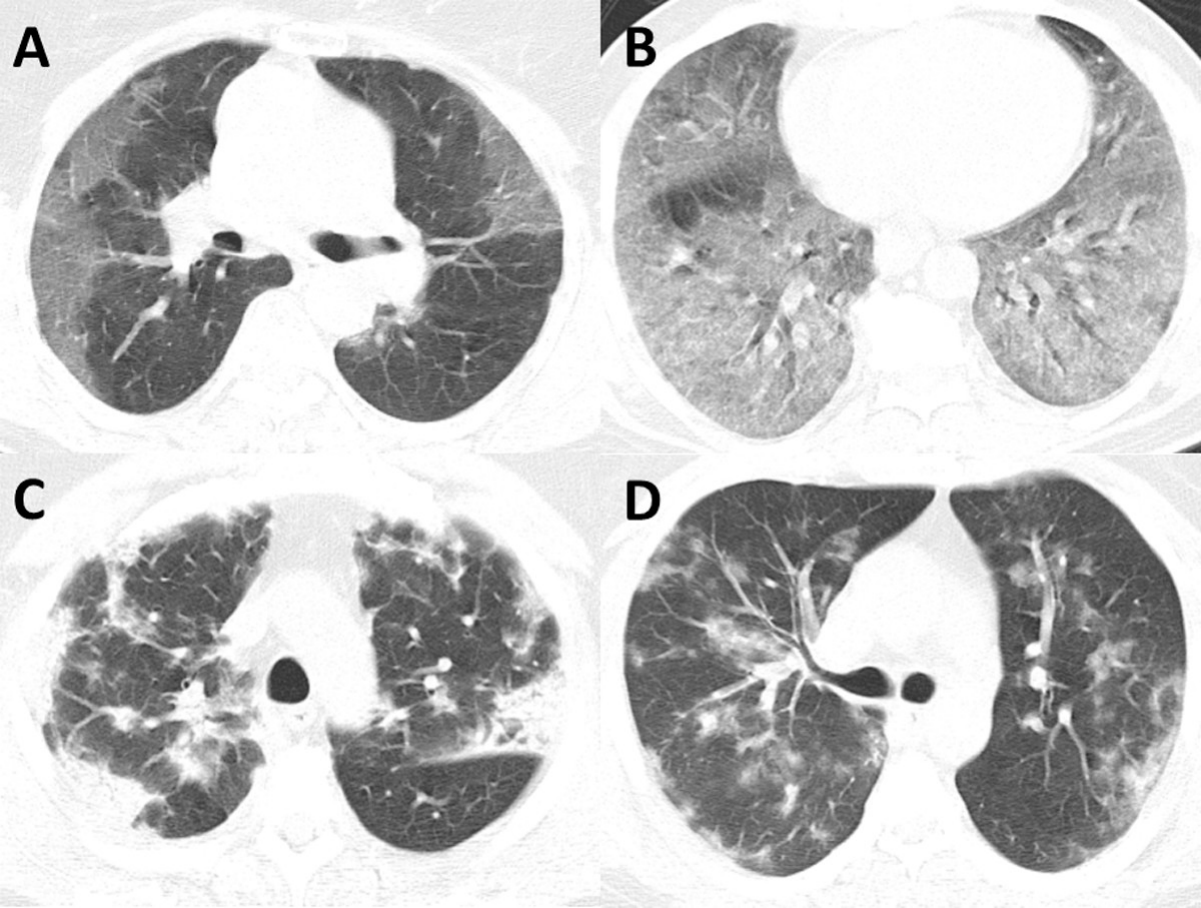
Examples of opacification patterns of COVID-19 on CT imaging. (A) Most common presentation of COVID-19 on CT imaging with multifocal peripheral ground-glass opacities. (B) Example of diffuse multi-lobar ground-glass opacities in COVID-19. (C) Example of consolidative and ground-glass opacities with both peripheral and central distribution. (D) Example of rare bilateral nodular consolidations seen in COVID-19.
